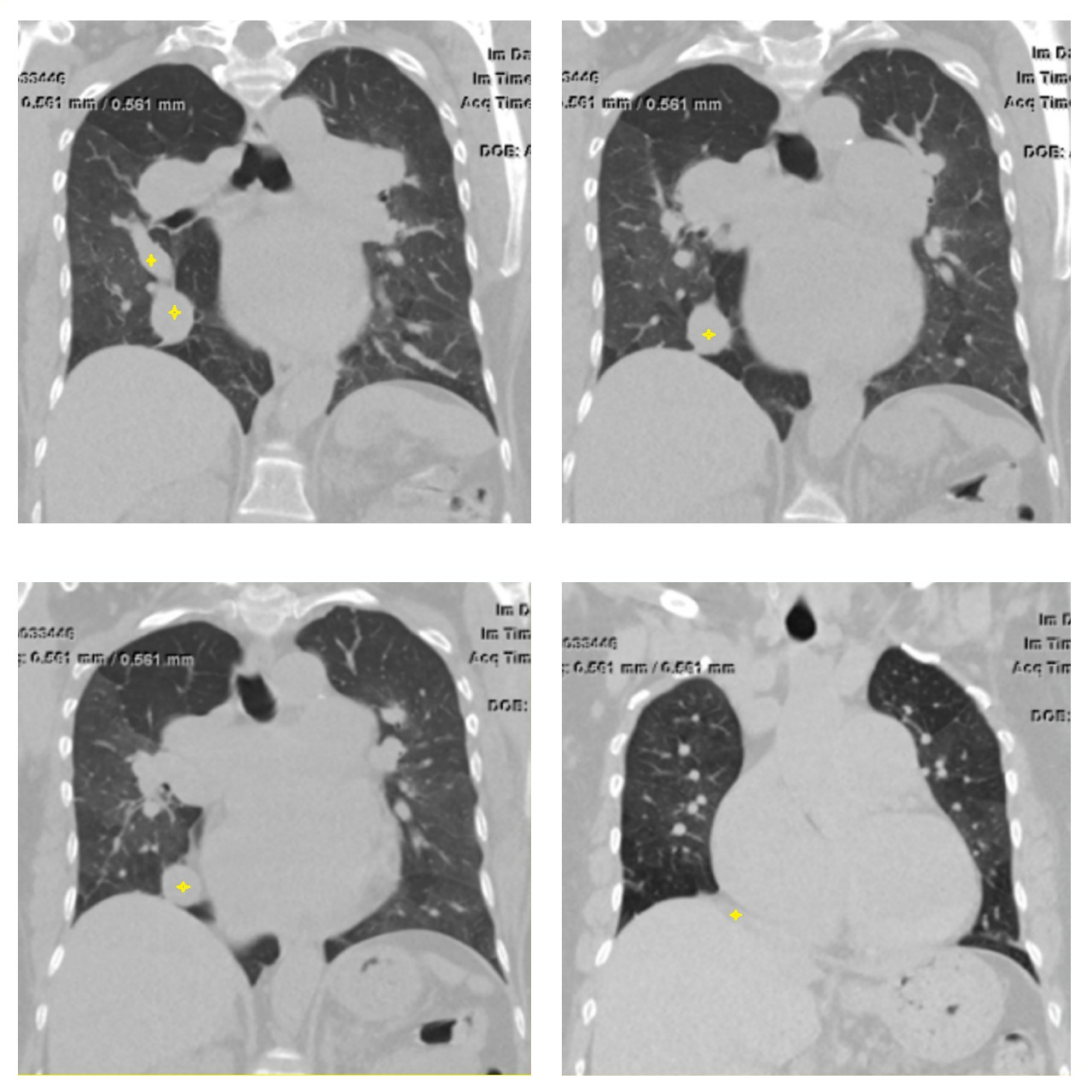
Computed tomography scan of chest (coronal reconstruction) demonstrating the Scimitar vein (marked by the yellow cross).
